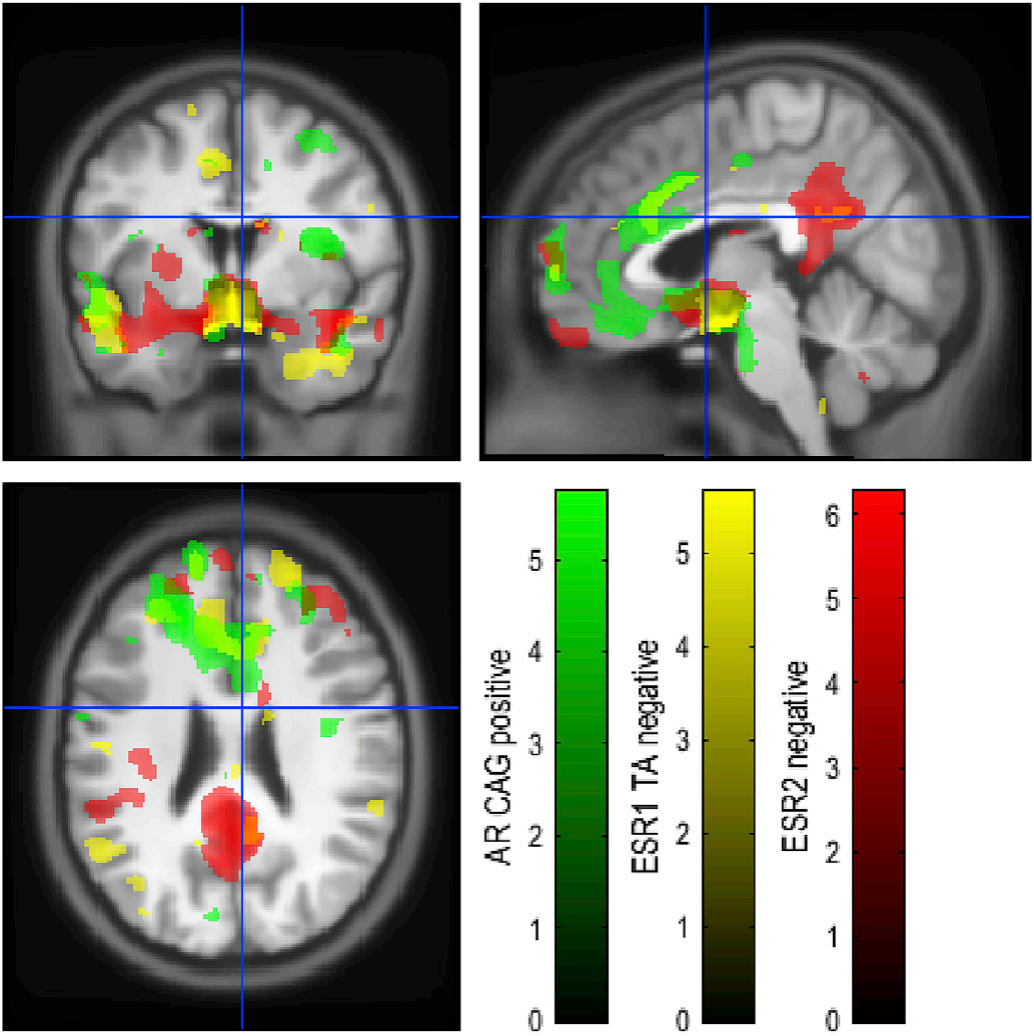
Regions associated with AR(CAG)n, ESR1(TA)n and ESR2(CA)n in GM volume. T-statistic maps thresholded at p ​< ​0.001 uncorrected were overlaid on the average of individual T1-weighted images warped together into MNI space.
